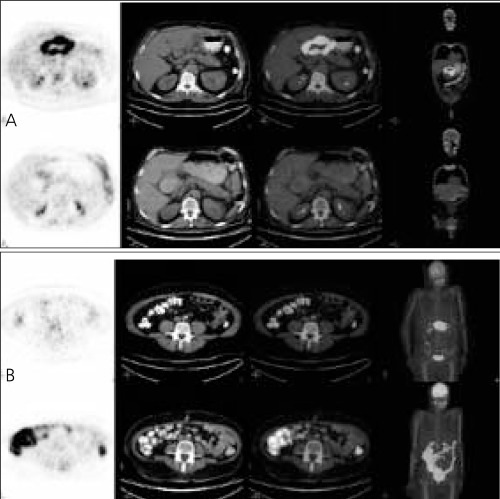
Selected images of pre-therapy and almost identical slices of posttherapyPET/CT seen in upper panel (1a); selected images of pre-therapyand corresponding slices of post-therapy PET/CT in the lower panel (1b)
